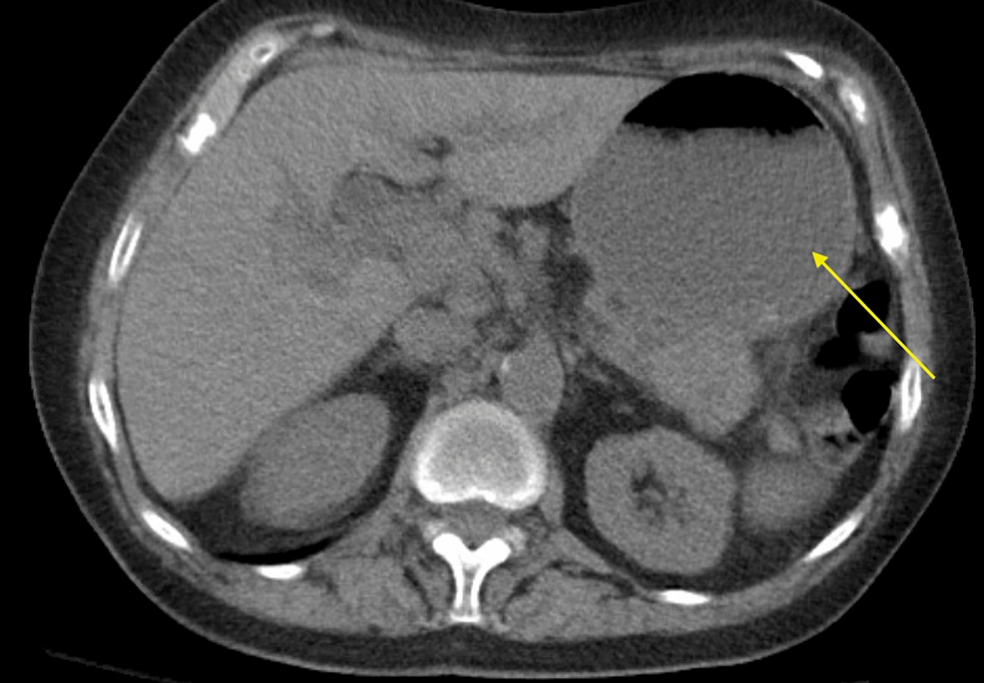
Computerized tomography (CT) of the abdomen and pelvis without contrast demonstrating gastric distension (yellow arrow) secondary to gastric outlet obstruction (GOO)
